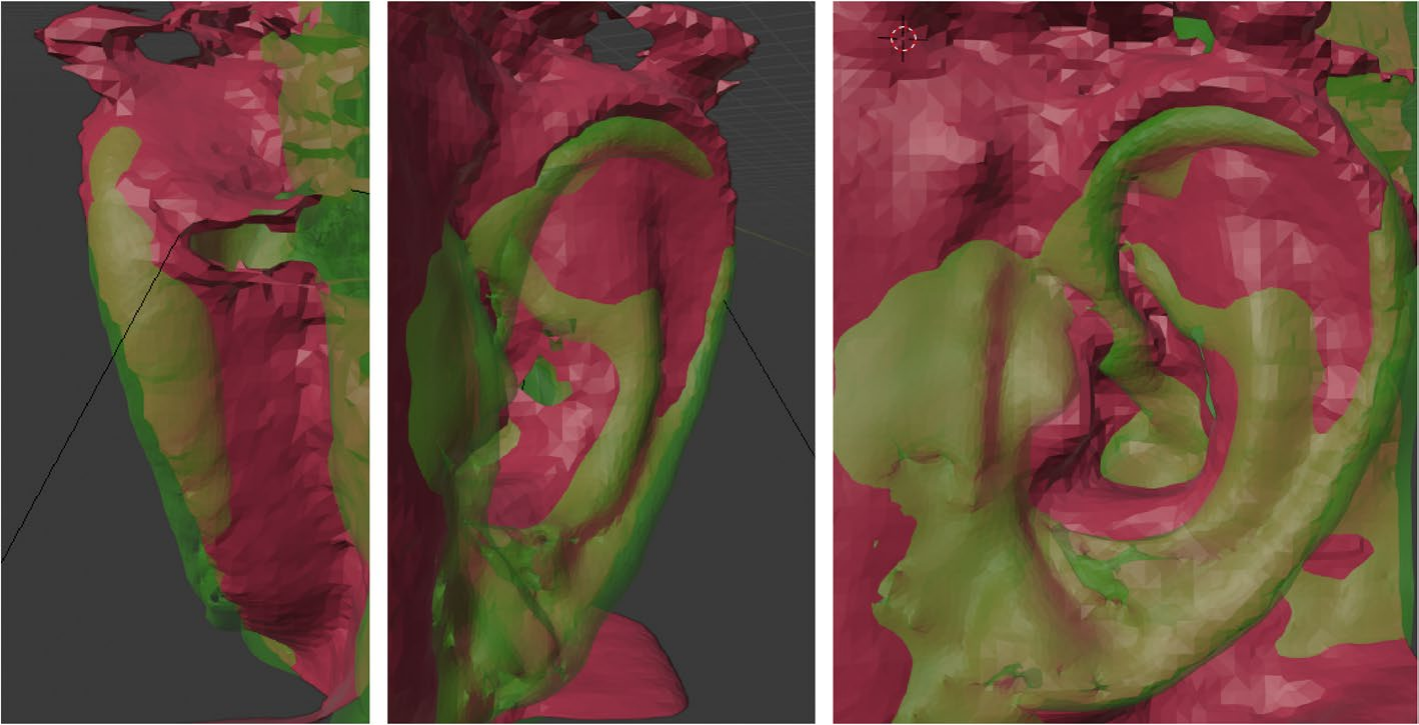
Image registration and image fusion of the MRI data (Fig. 3) and optical data (Fig. 6, left) obtained from same person (author TW). Left side: posterior-anterior view; center: anterior-posterior view; right side: side-view. ‘Brown’ indicates data from the optical scanner, while ‘green’ represents data from the MRI. The MRI data has a much higher resolution quality and is much smoother than the data obtained from the optical scanner
